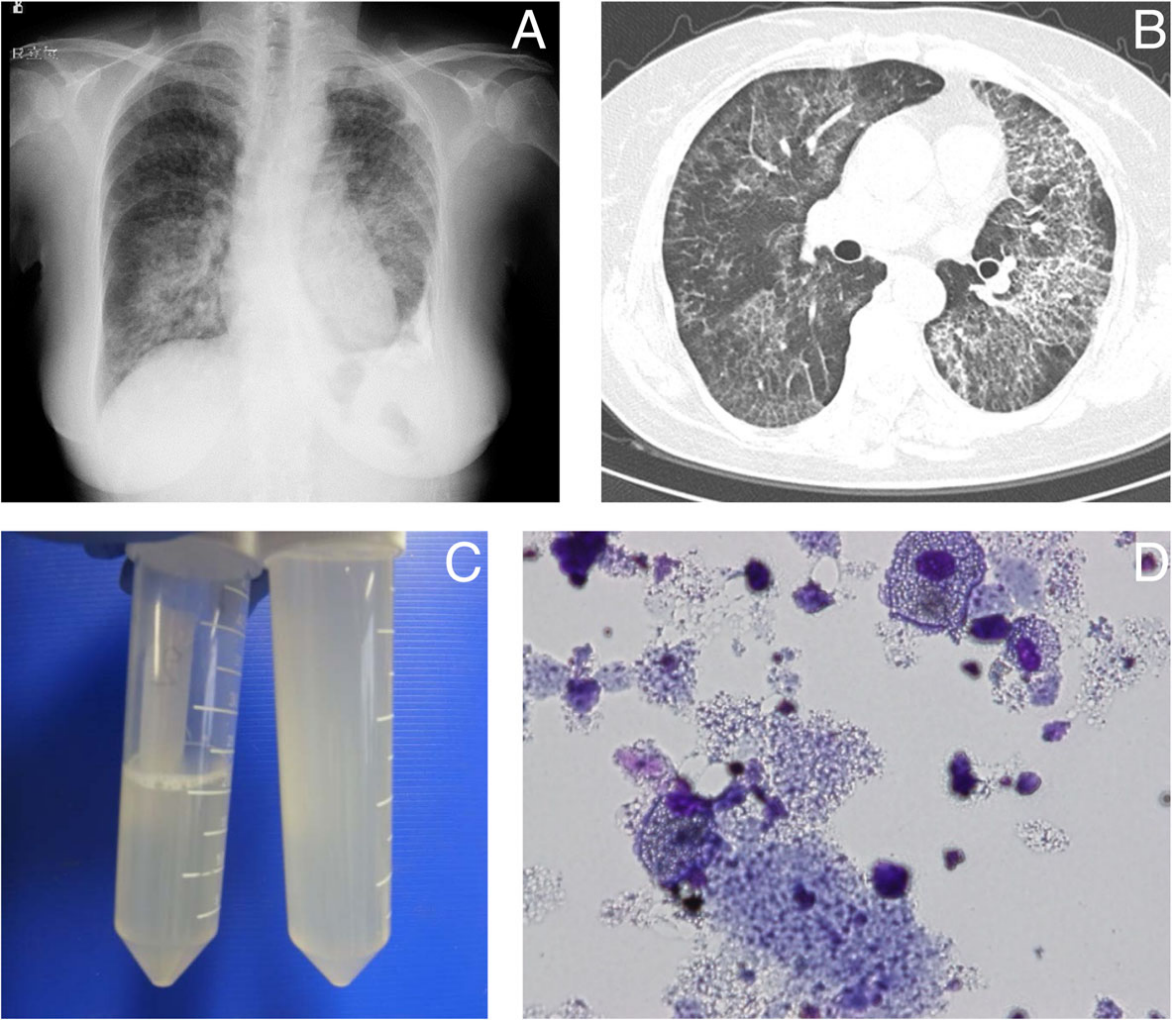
a Chest radiogram of the patient. b High-resolution computed tomogram of the patient. c BALF presented a milky appearance with lymphocytosis (26%). d Foamy macrophages and amorphous materials in BALF of the patient
